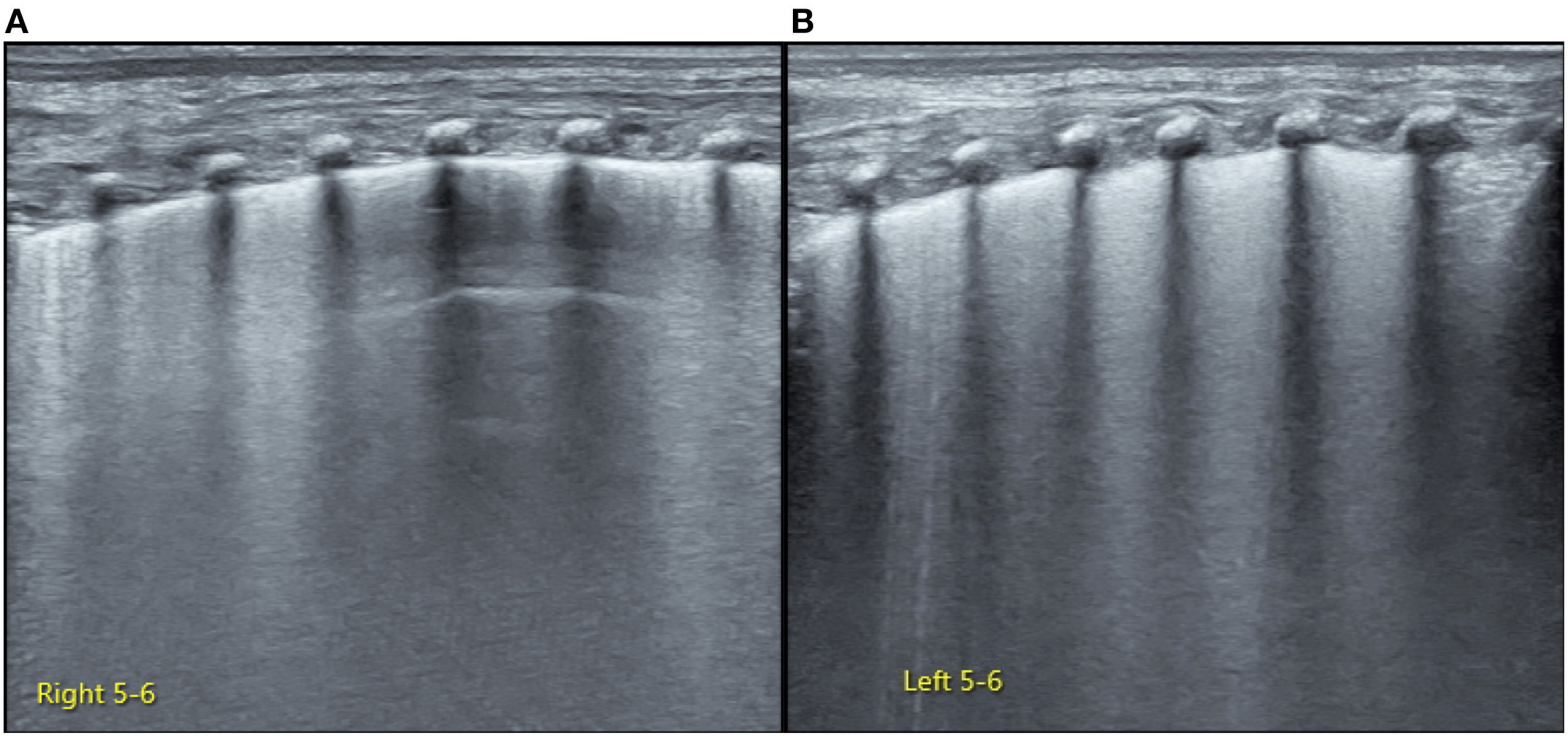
Lung ultrasonography (LUS) examination after thoracocentesis. LUS examination shows resolution of atelectasis in bilateral lungs after pleural puncture therapy. The right upper-lung and lower-lung field mainly present as confluent B-line (but the A-line can be found in the middle-lung field), which suggests some degree of lung edema (A). The left lung in the last intercostal space reveals snowflake signs while the remaining intercostal spaces present as ground-glass opacities, which are characteristic of respiratory distress syndrome (RDS) (6, 7) (B).
